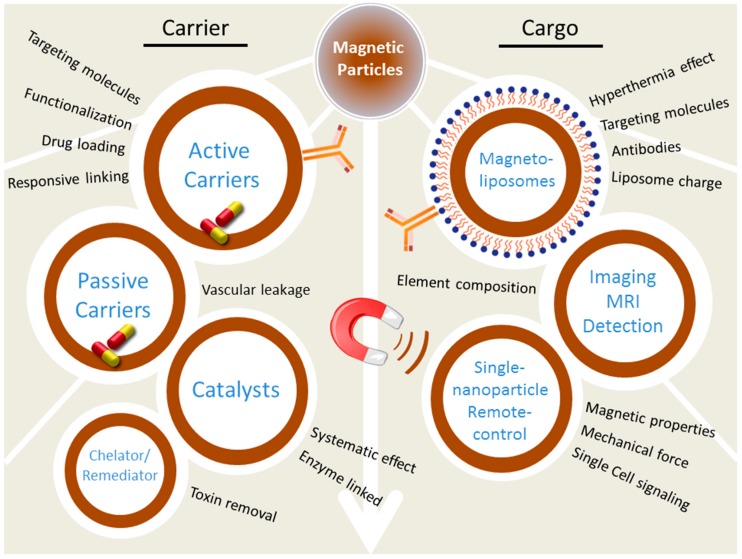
The scheme of magnetic particles utilization. MRI: magnetic resonance imaging.
